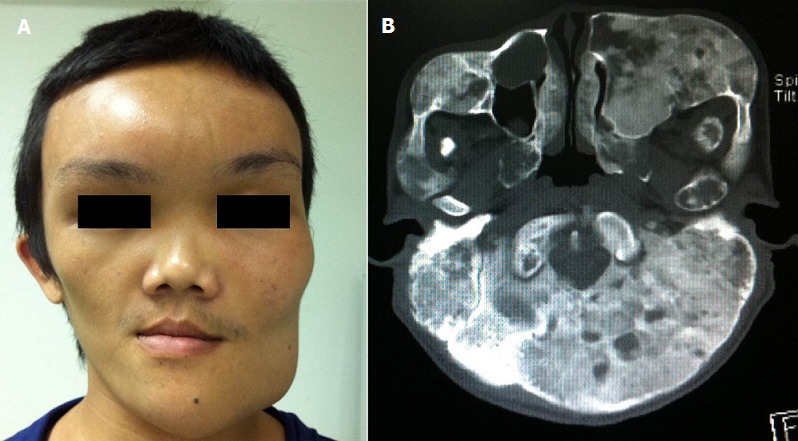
A) the patient picture. Note asymmetrical facial figure especially the forehead and left side; B) a CT scan (axial view). Multiple expansile lesions with a heterogeneous pattern, including radiolucency interspersed with ground-glass opacity of craniofacial bones
